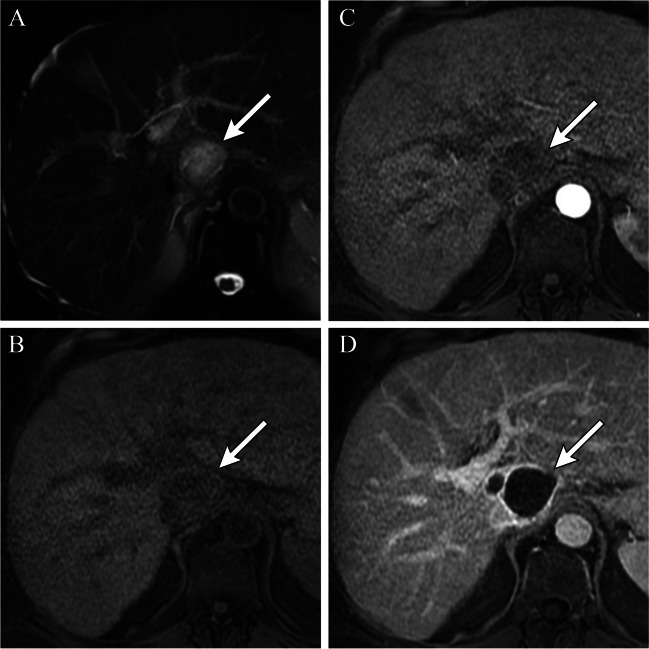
67-year-old male with HCV cirrhosis presenting with LR 5 HCC within the caudate lobe (images not shown). MRI post cTACE with lipiodol shows mild T2 hyperintense signal within the treated lesion (A). T1 pre-contrast fat-saturated images demonstrate hypointense signal within the treated lesion (B) with no post-contrast enhancement on arterial (C) or portal venous phase of imaging (D). Smooth perilesional rim of enhancement is consistent with expected post-treatment imaging findings (D). This lesion is categorized as mRECIST CR or LR-TR nonviable
